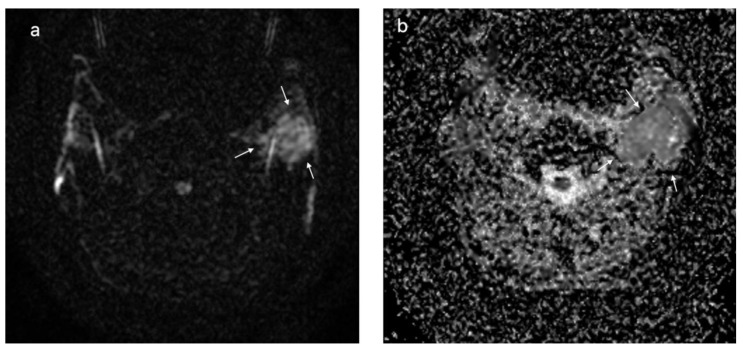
Pleomorphic adenoma located at the level of the left parotid gland (white arrows); DWI sequences (a) with ADC map (b) showing a value of 1.6 × 10^−^^3^ mm^2^/s.
